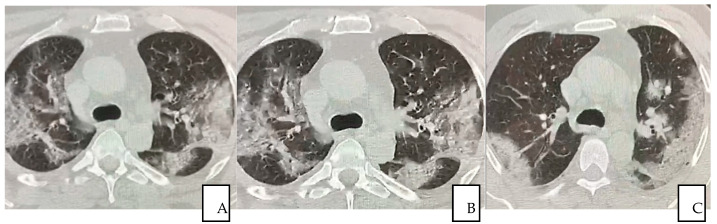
(A–C) A 56-year-old man from “SG Moscati Hospital”, Taranto, presented with fever, cough, and chest pain. Pulmonary HRCT at presentation on 3 March 2020 showed extensive peripheral predominant ground-glass opacities in both upper lobes (A); On 26 March 2020, a follow-up HRCT showed similar anomalous ground glass opacities (B) HRCT obtained on 14 May 2020 showed organizing changes with decrease in extent of the ground-glass opacities and increasing parenchymal consolidations (C).
